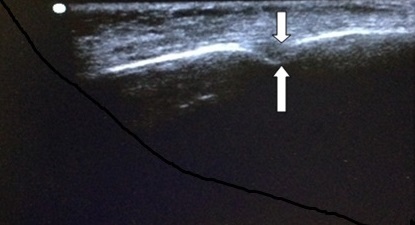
Ultrasound image of the mental foramen (between white arrows); the mental foramen is easily identified as a break in the continuity of the bone in the vicinity just below the corner of the mouth
